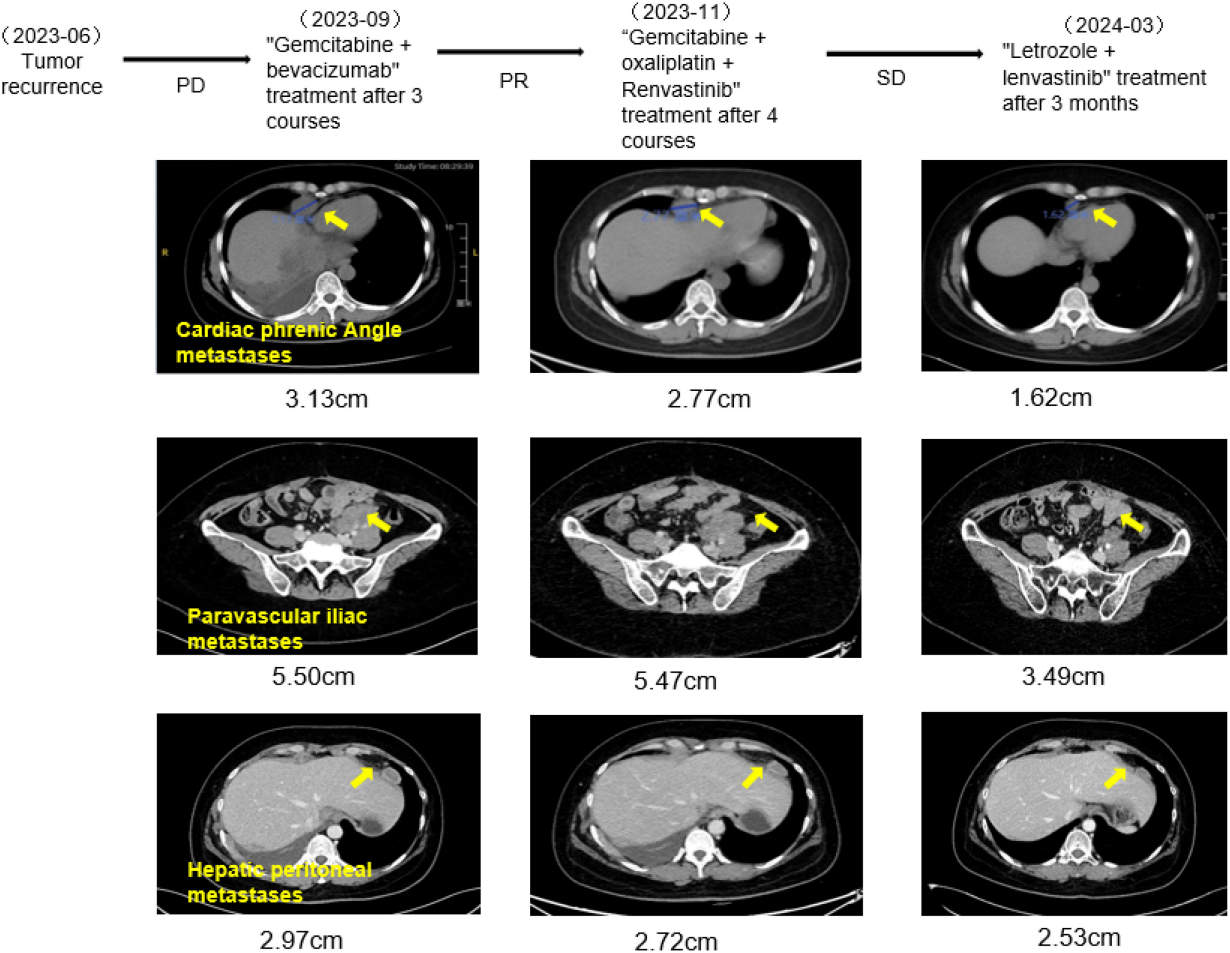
CT examination of the patient (the trend of lesion change with the change of treatment regimen).
